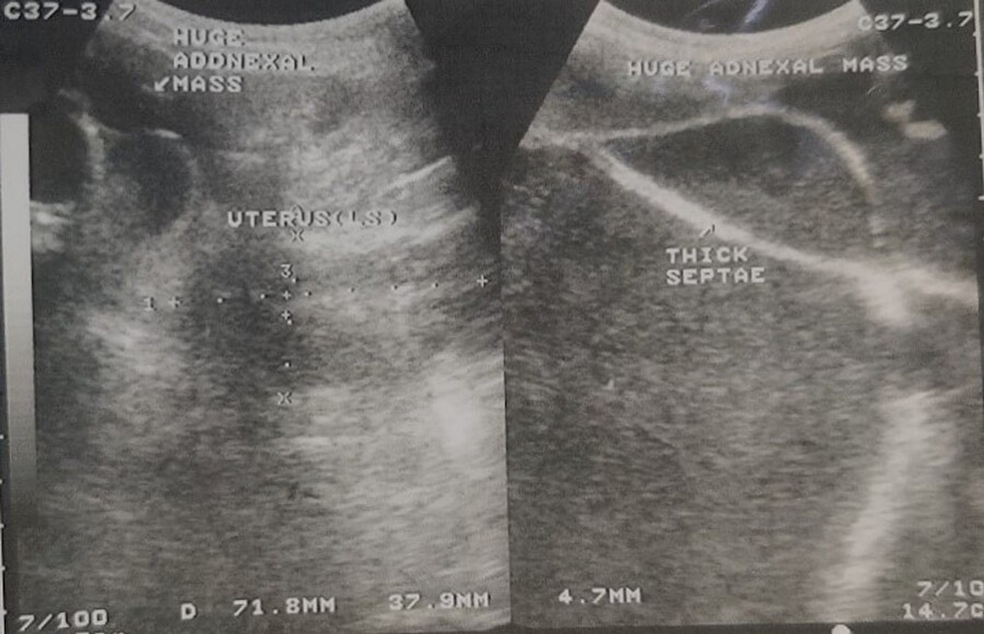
Greyscale transabdominal ultrasonographic picture showing multiloculated, solid cystic mass with thick septae (left and right)
